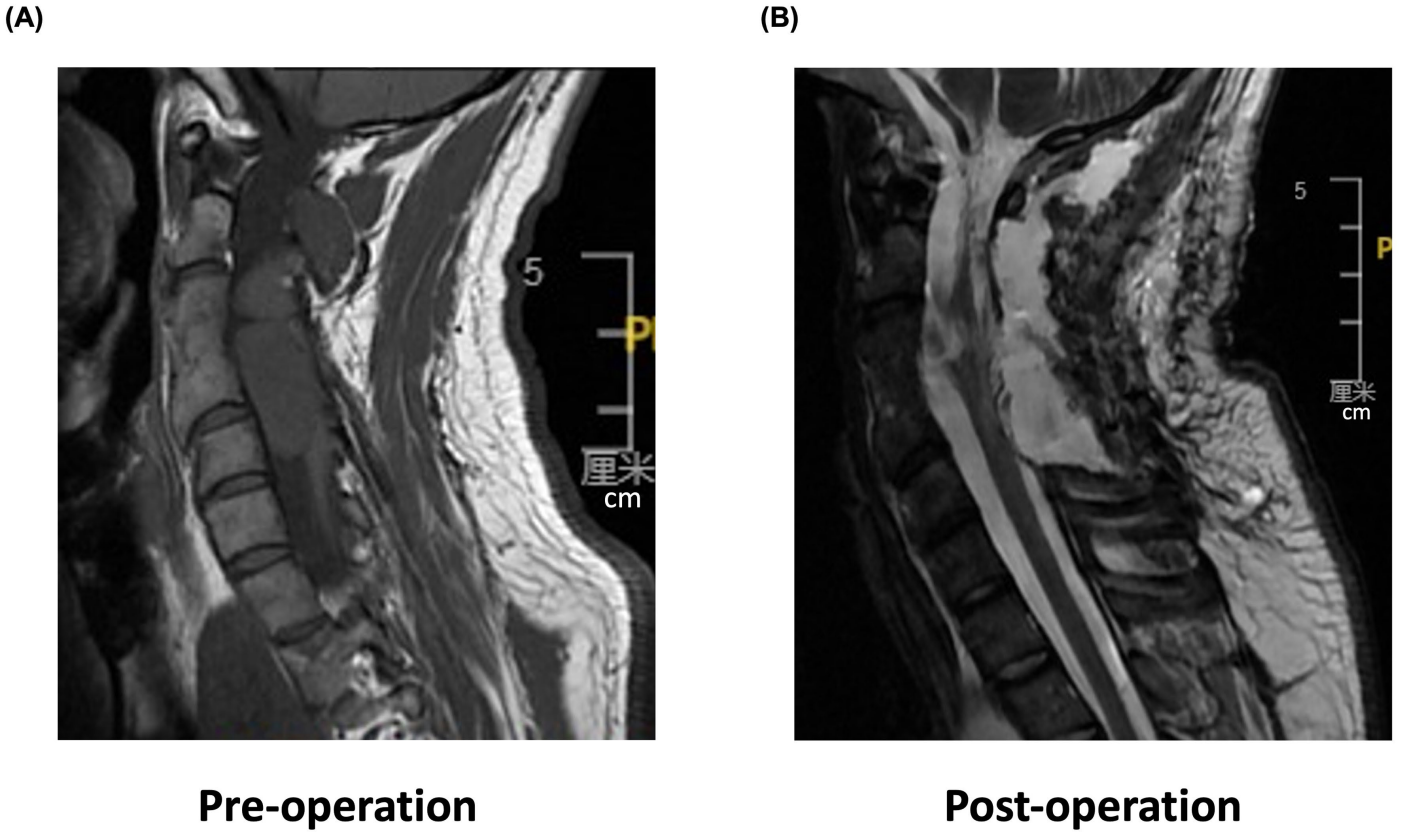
Cervical spine magnetic resonance imaging (MRI). (A) Pre-operation sagittal T2-weighted MRI shows a high T2 signal with spinal cord compression in C2–C5 segments. (B) Post-operation sagittal MRI reveals morphological changes in C2–C4 segments of the spinal cord with mixed signal intensity and irregular edges.
